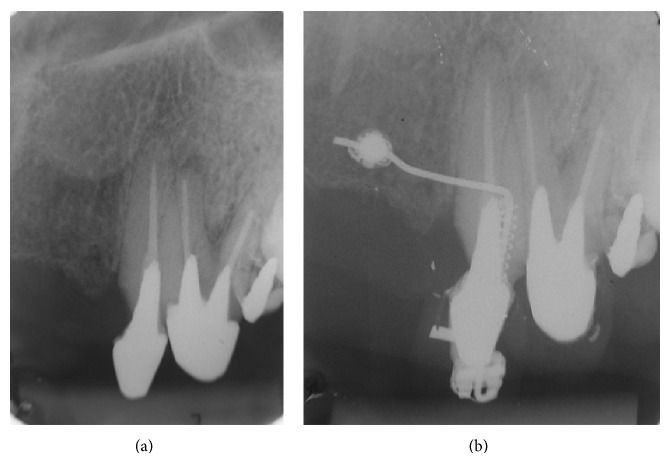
Initial and final radiographs.
